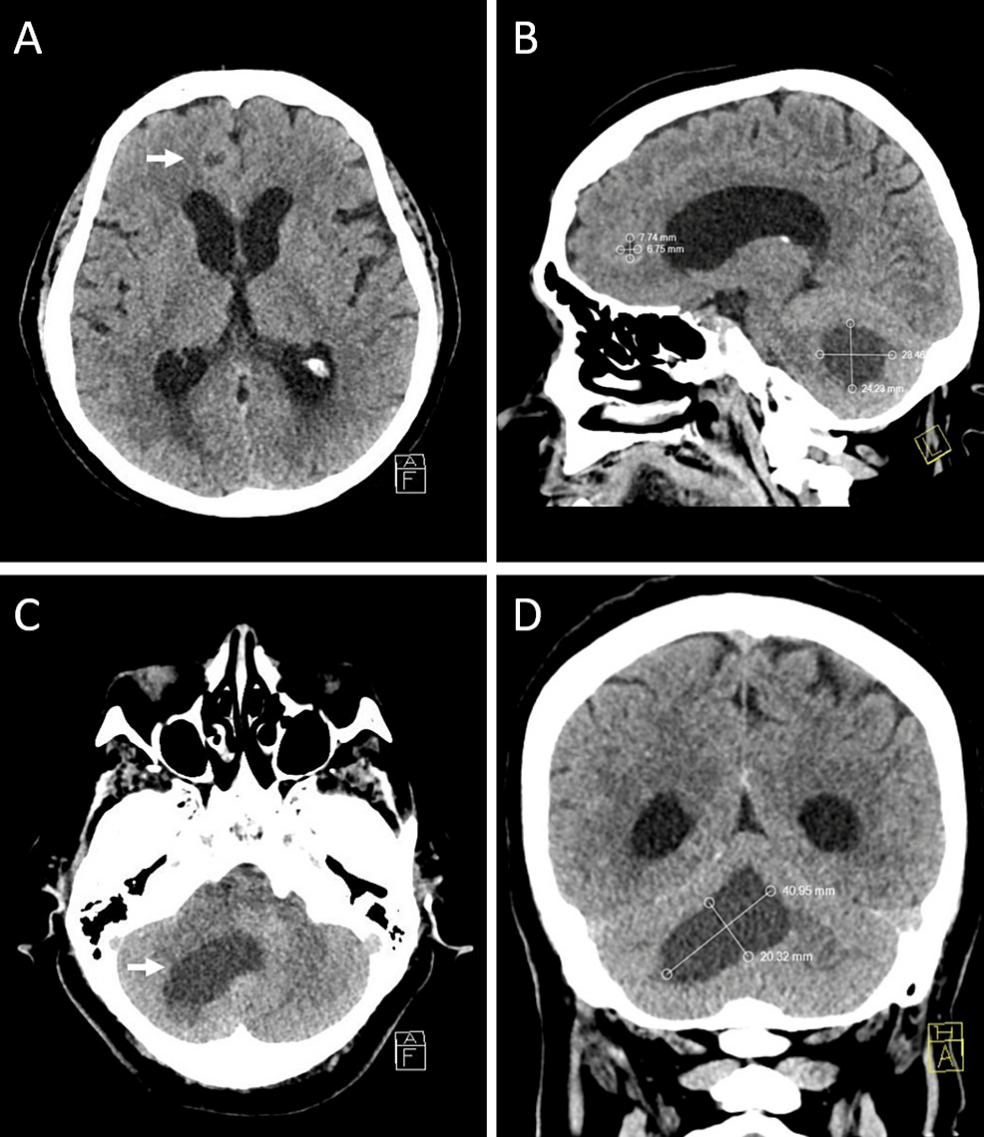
CT of metastatic brain disease (A and C) Axial views: metastatic lesion in the left frontal lobe and the right cerebellar hemisphere with perifocal cytogenic edema. (B) Sagittal view: one metastatic lesion in the frontal lobe measuring 7.74x6.75 mm and another in the right cerebellar hemisphere measuring 28.46x24.23 mm at their largest sizes. (D) Coronal view: an extensive metastatic lesion in the right cerebellar hemisphere measuring 40.95x20.32 mm at its largest, compressing the inferior longitudinal CT: computed tomography
